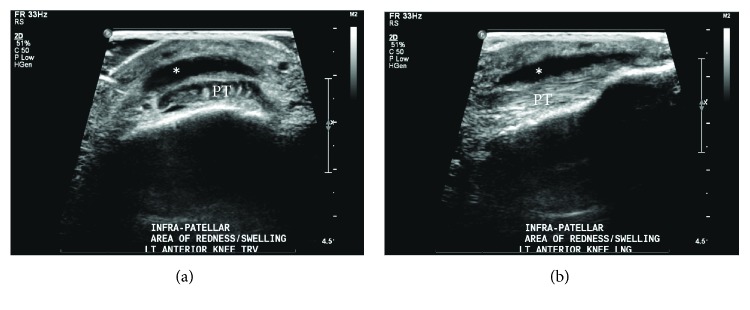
Ultrasound imaging of the left infrapatellar bursa in both cross-sectional (short axis) (a) and longitudinal (long axis) (b) views. ^∗^Infrapatellar bursa is enlarged. The patellar tendon (PT) looks normal.
